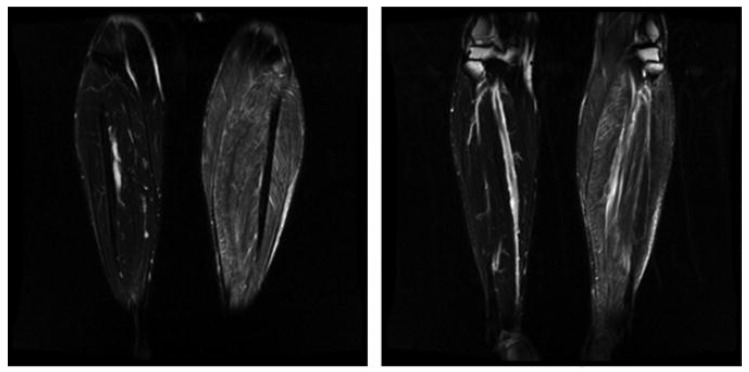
MRI of the lower limbs in the case of toxic myopathy. The T2 images show asymmetric involvement affecting only the left lower limb. Had a muscle biopsy been taken from the right gastrocnemius, the pathologic tissue would have been missed [4] © 2012 The Authors. Physical Medicine and Rehabilitation Clinics of North America by Elsevier.
